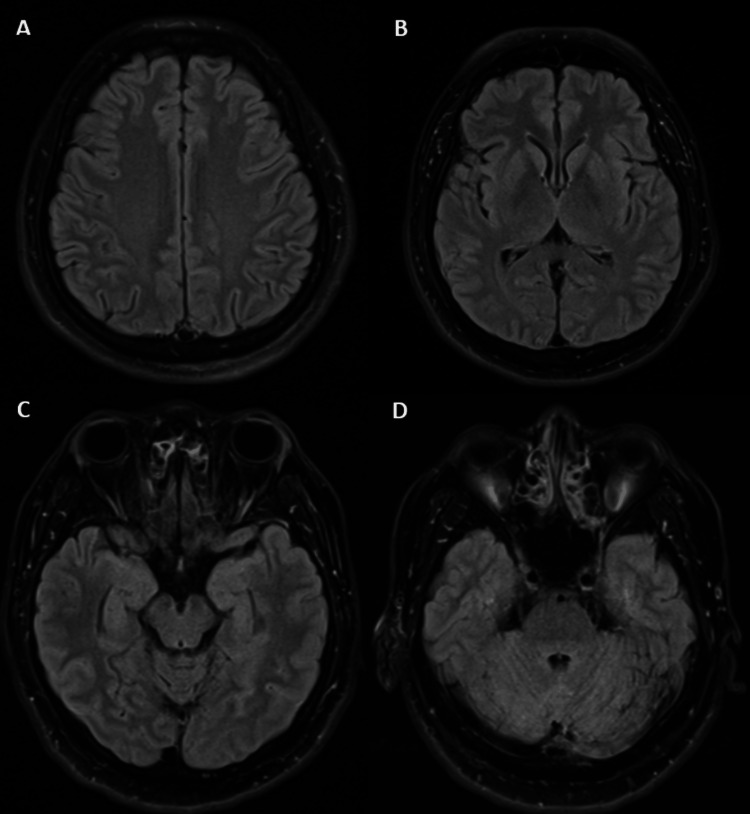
MRI T2-FLAIR of the patient did not show any significant limbic system changes. (A-D) No significant abnormalities were detected. MRI T2-FLAIR: magnetic resonance imaging T2-weighted fluid-attenuated inversion recovery
